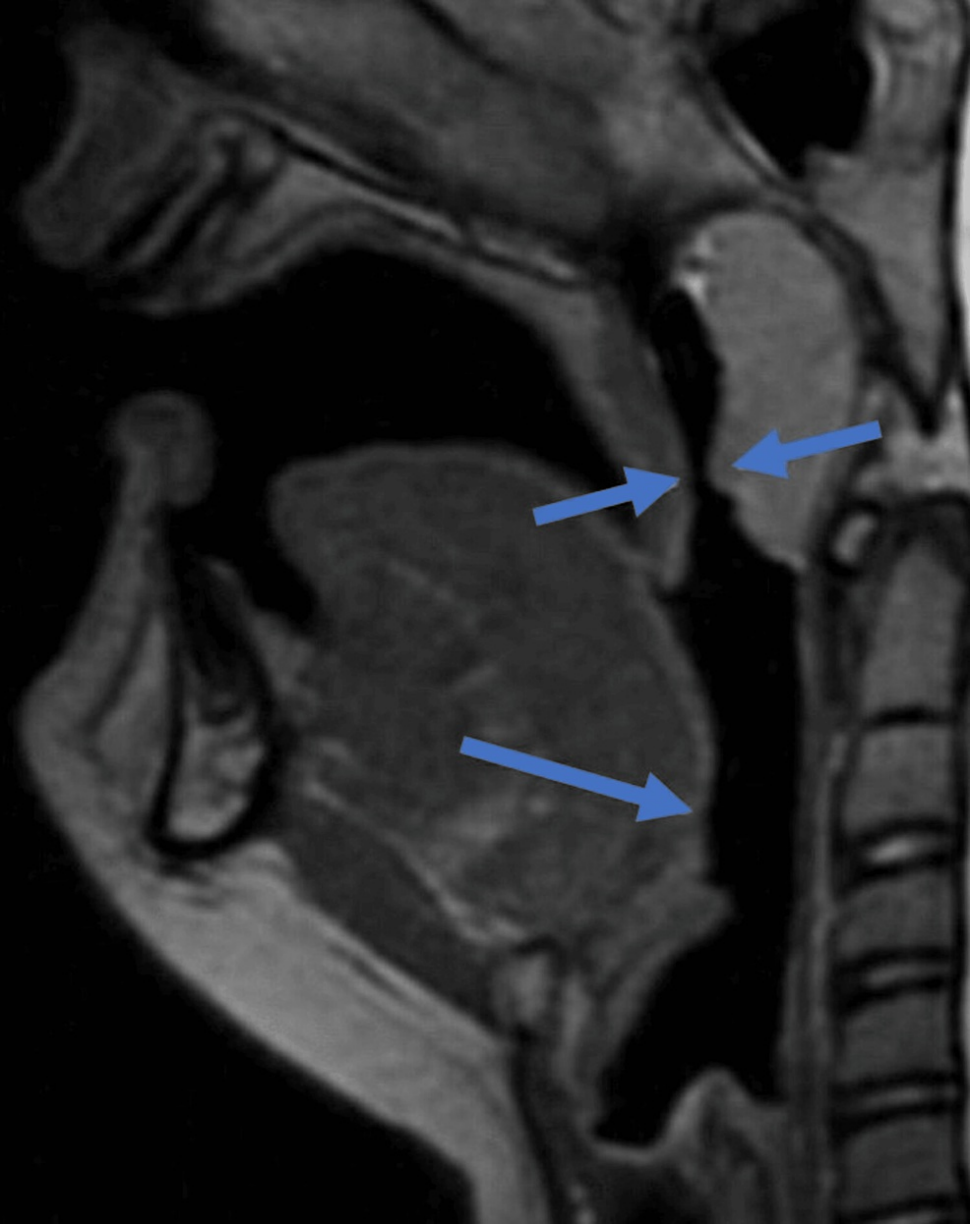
MRI- Sagittal section revealed long uvula that constricts the airway spaces
